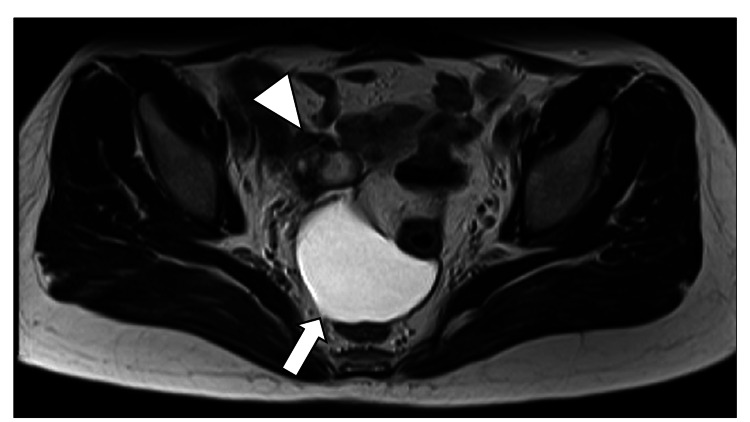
Magnetic resonance imaging of the inclusion cyst (T2-weighted) before transvaginal oocyte retrieval. An inclusion cyst (arrow) adjacent to the right ovary (arrowhead) appeared.
